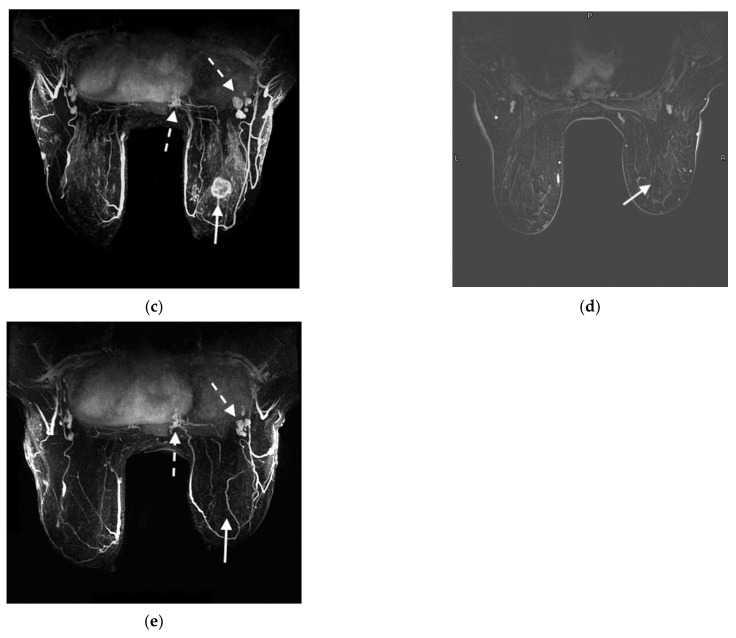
47-year-old woman with a right breast invasive ductal carcinoma. (a) Prechemotherapy axial contrast-enhanced T1-weighted fat-suppressed MRI shows a 2.7 × 2.5 × 2.2 cm irregular mass with a thick rim of enhancement in the upper outer right breast (arrow), compatible with the known invasive ductal carcinoma. (b) Prechemotherapy axial contrast-enhanced T1-weighted fat-suppressed MRI shows an area of non-mass-like enhancement compatible with DCIS (edges demarcated by (*) located posterior to the mass (arrow). (c) Prechemotherapy maximum intensity projection image demonstrates a right breast mass (arrow) with associated enhancing right axillary and intramammary lymphadenopathy (dashed arrows), compatible with metastatic disease. (d) Postchemotherapy contrast-enhanced T1-weighted fat-suppressed MRI shows resolution of the right breast mass. Susceptibility artifact from the biopsy clip is seen in the tumor bed (arrow). The area of non-mass-like enhancement has also resolved (not shown). (e) Postchemotherapy maximum intensity projection image demonstrates resolution of the previously seen large right breast mass (solid arrow in tumor bed). The right axillary and intramammary lymph nodes are redemonstrated but have decreased in size (dashed arrow).
